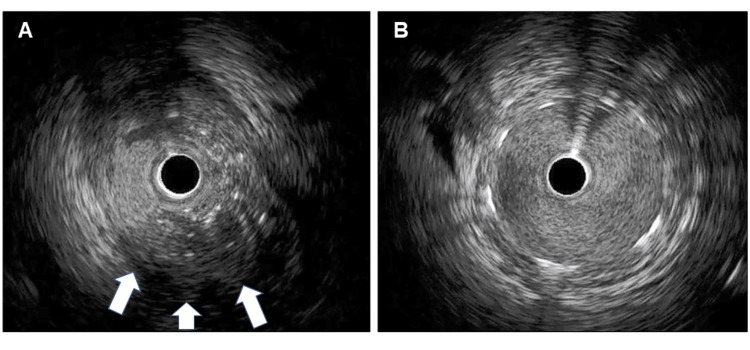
Intravascular ultrasound images (A) A low echoic mass including calcified granules (arrows). (B) A well-dilated drug-eluting stent without protrusion.
